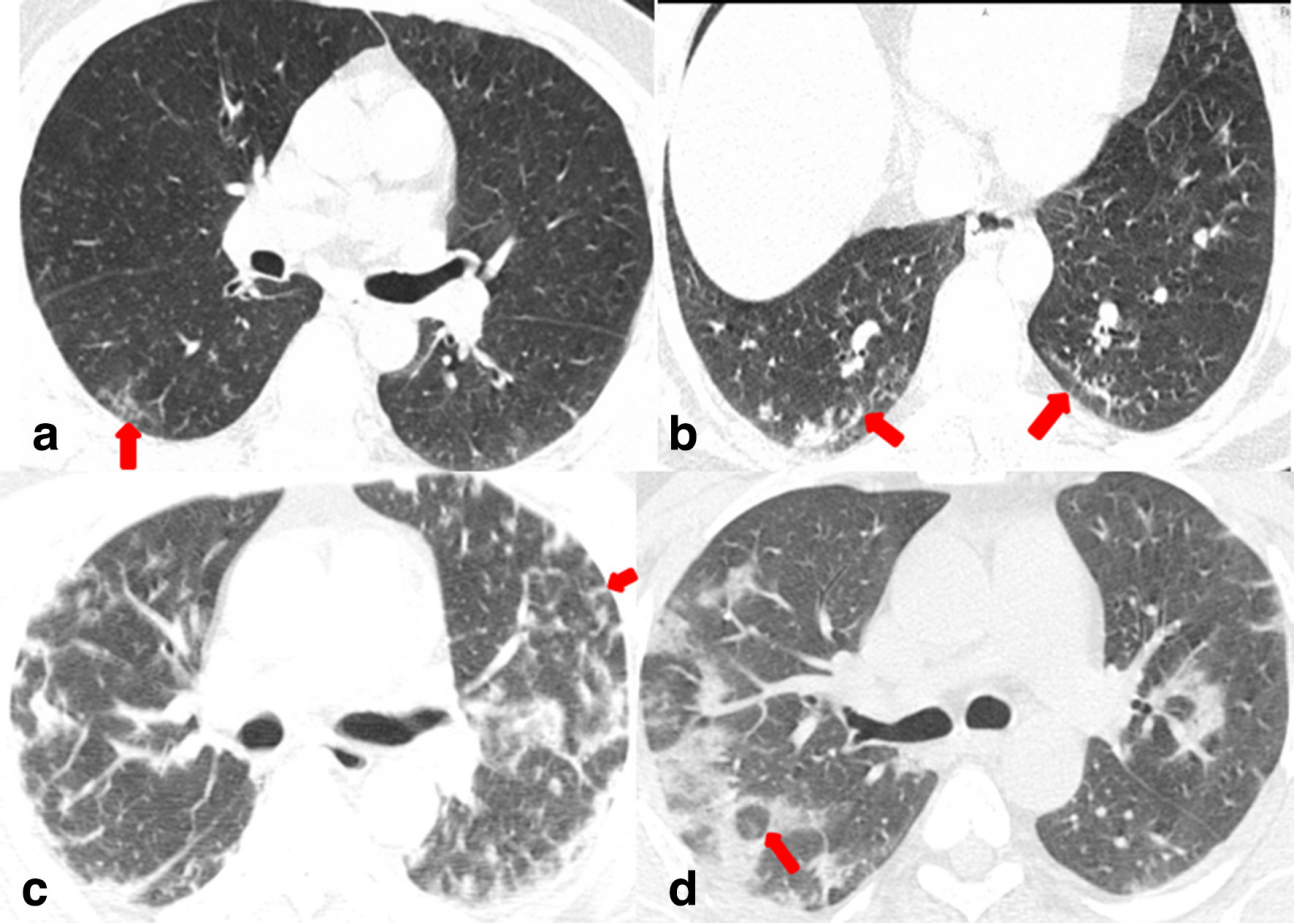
Non-contrast axial chest CT images in lung window settings in four different clinically stable (routine ward patients) COVID-19 positive patients: (a) 50-year-old male showing subpleural reticulations (arrow); (b) 56-year-old male showing subpleural curvilinear lines (arrows); (c) 61-year-old male showing multiple bilateral linear and reticular opacities in an arc pattern resembling peri-lobular opacities (arrow) typical of organizing pneumonia and (d) 49-year-old male showing bilateral multiple organizing consolidations with central lucency suggestive of reverse halo (atoll) sign (arrow).
